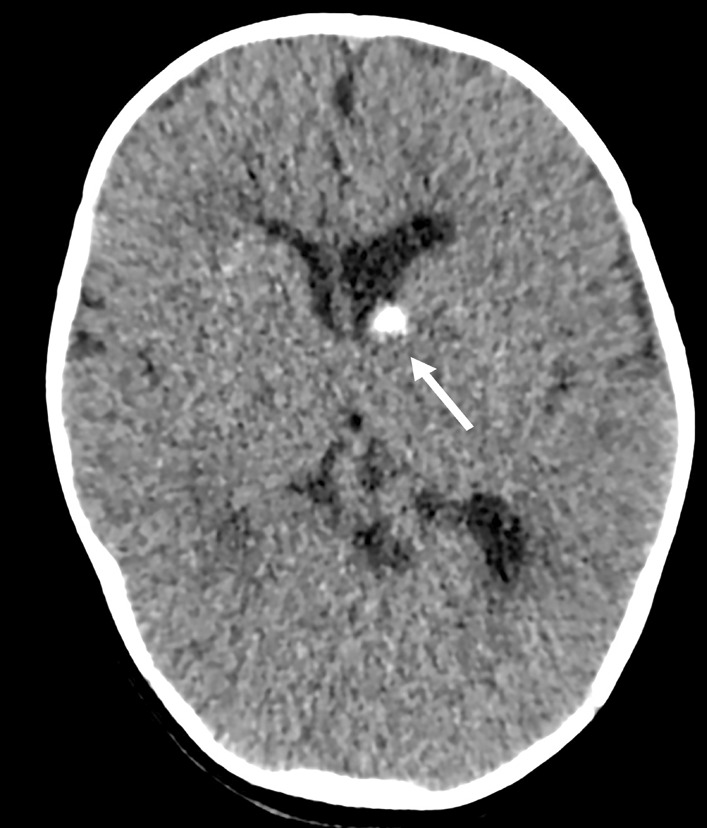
Case 11: Figure 1. Axial brain CT image. Calcified lesion is seen adjacent to left lateral ventricle
